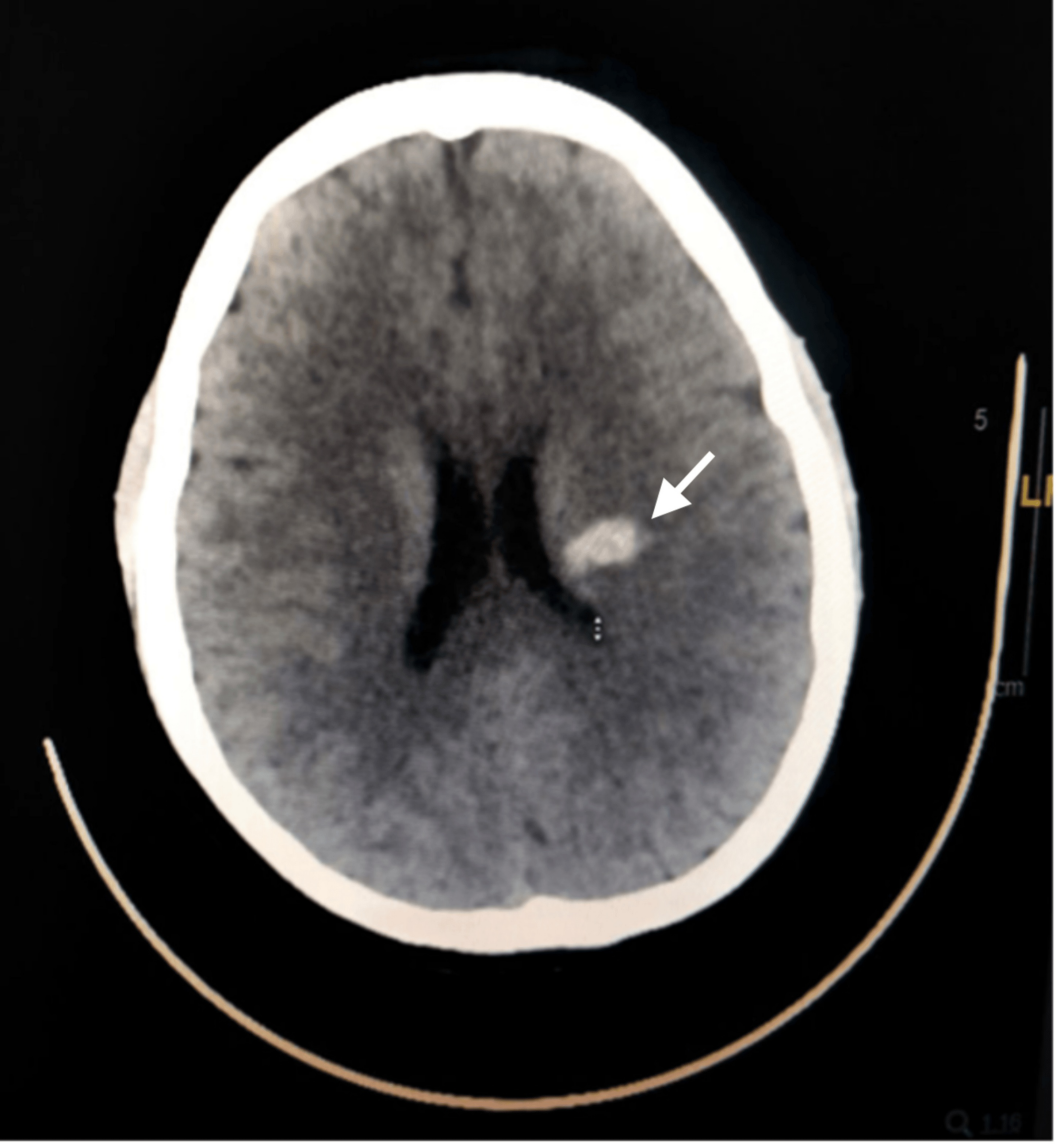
Axial CT brain depicting the presence of a left sided high parietal hematoma
